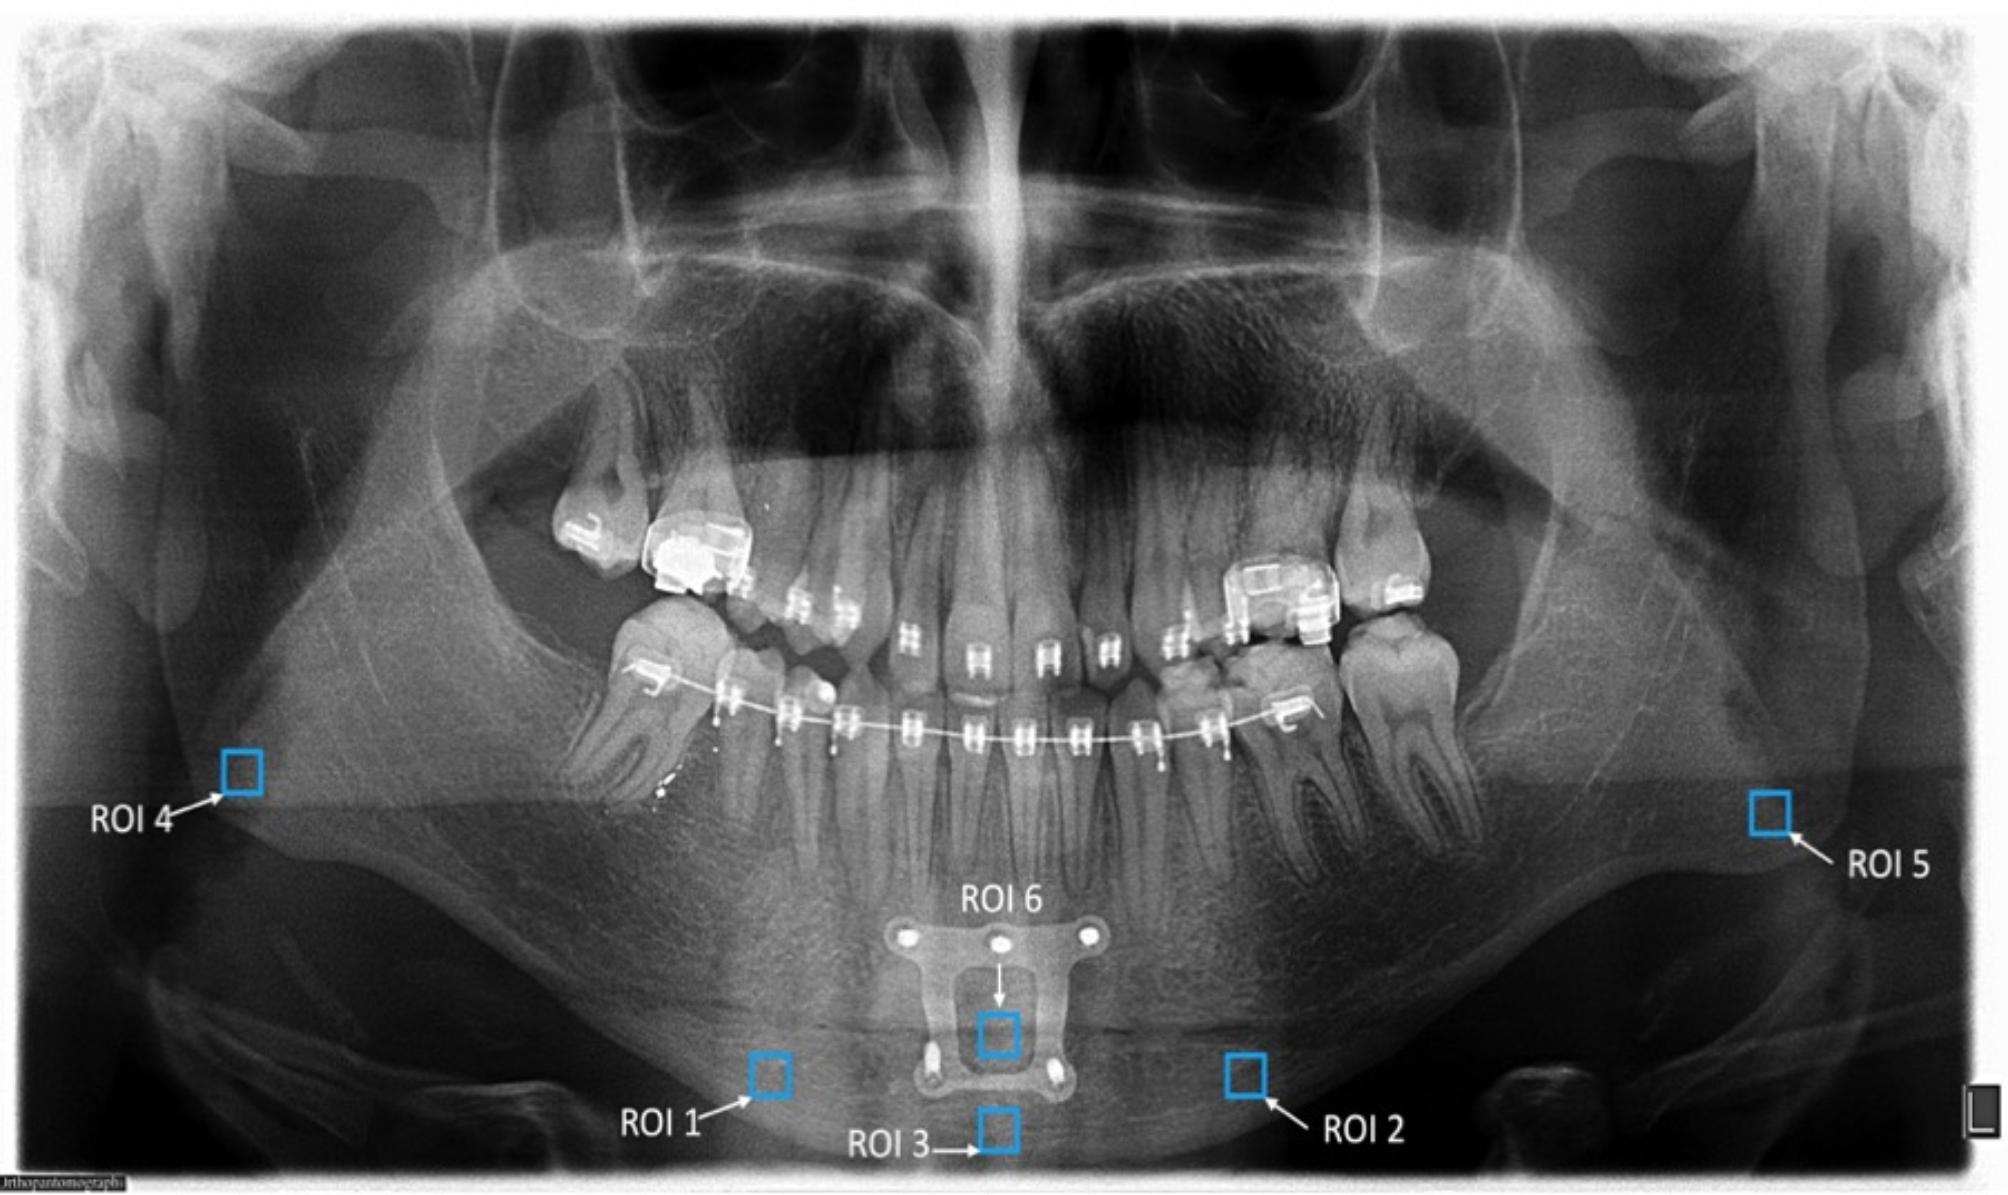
The region of interests (ROIs) was obtained from the panoramic radiographs, a square of 50 × 50 pixels. ROI 1: right region of the separated genial segment. ROI 2: left region of the separated genial segment. ROI 3: middle region of the separated genial segment. ROI 4: region selected in the right mandibular angulus. ROI 5: selected region in left mandibular angulus. ROI 6: healing region between the separated genial segment and mandibular corpus
